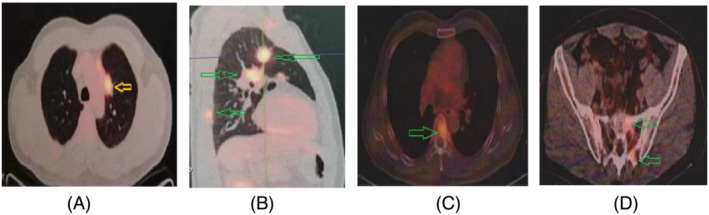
Positron Emission Tomography/CT scan on January 28, 2021. Positron Emission Tomography/CT scan showed a hypermetabolic focus in left lung (SUVmax = 8.7, A) with multiple lymphatic metastases of hilar and mediastinum (B) and multiple bone metastases (B–D, ribs, vertebra, and pelvic bones). Positron Emission Tomography/CT revealed the lung cancer with systemic metastases.
